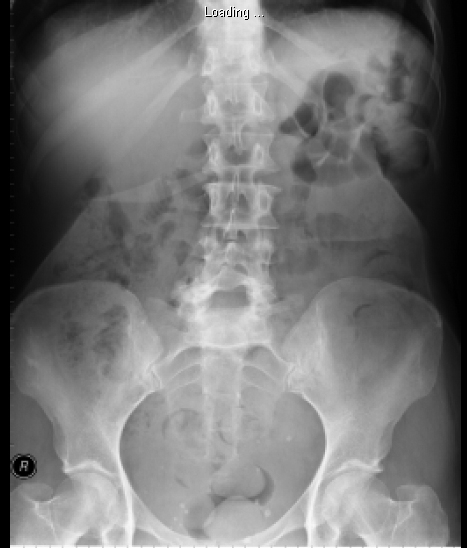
Plain abdominal x-ray showing the foreign body in the right upper quadrant of abdomen.
